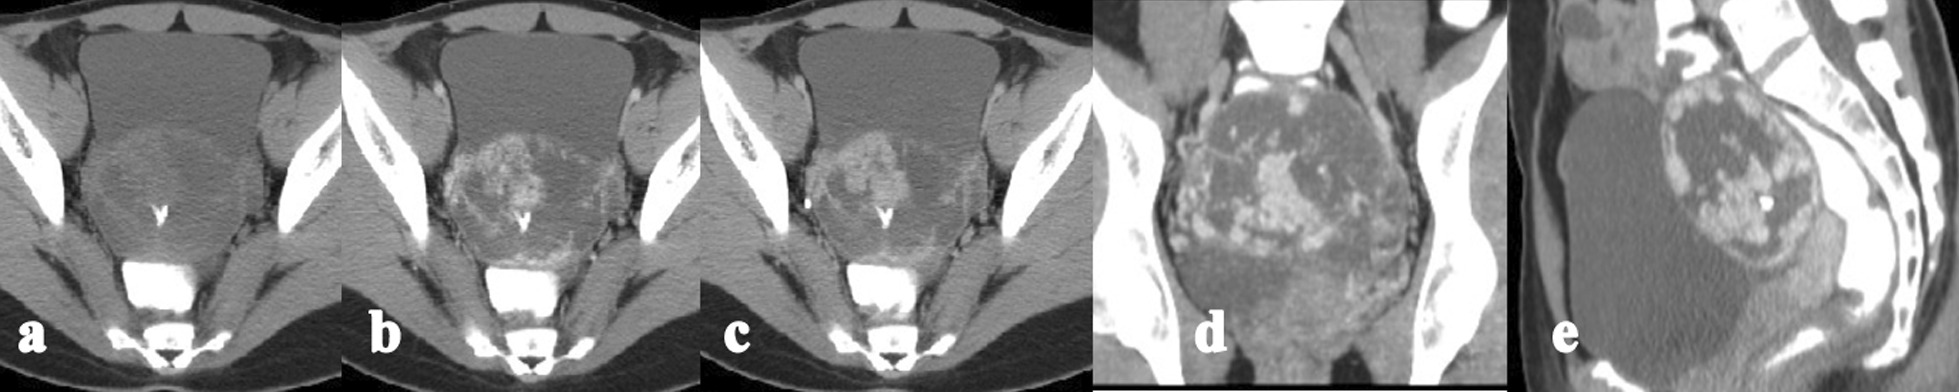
Case CT01. Axial plain CT scan (a) showing a round and well-defined heterogenous mass, and a patchy calcification in the center of the mass; b–e dynamic enhancement CT scan showing early-fast sustained enhancement
